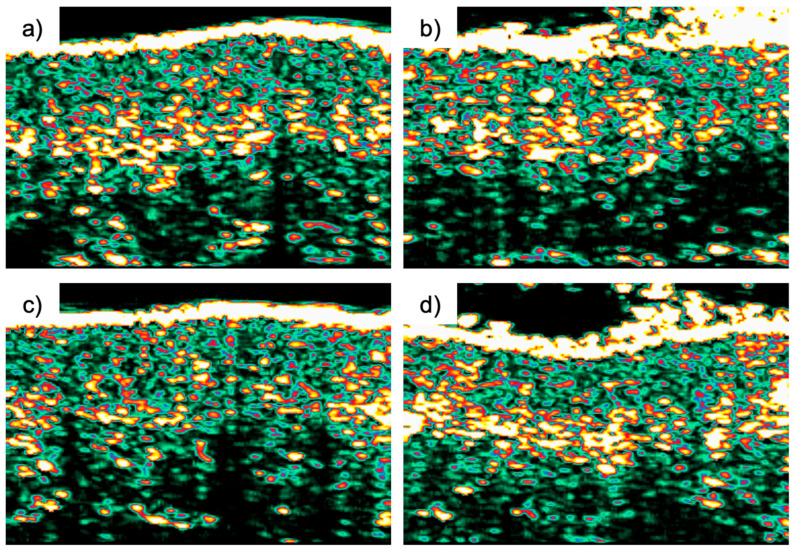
Ultrasound measurements in the tragus regions before and after filler injection assessing dermal thickness. (a) Dermal ultrasound before Rennova^®^ Diamond Intense injection, (b) dermal ultrasound after Rennova^®^ Diamond Intense injection, (c) dermal ultrasound before Radiesse^®^ injection, and (d) dermal ultrasound after Radiesse^®^ injection. The images were acquired using high-frequency ultrasonography (DermaLab^®^ Combo-4), which captures variations in skin density and echogenicity. The brighter, more reflective upper layers correspond to the dermis, while the darker areas below represent subcutaneous tissue. Increases in dermal thickness and echogenic signal after injection indicate enhanced structural density and collagen biostimulation, as shown by the more prominent and broader bright zones in panels (b,d) compared to their respective baseline images (a,c).
